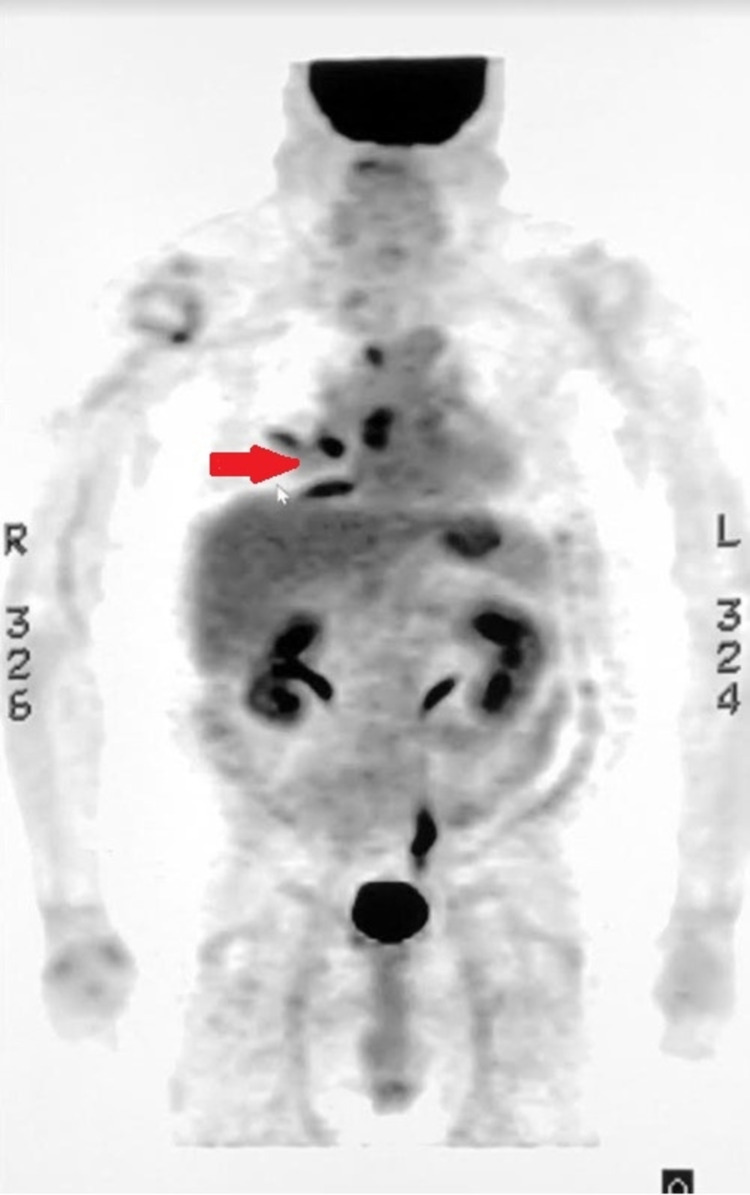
PET/CT scan which showed a large FDG avid right hilar and mediastinal lymph nodes with right paratracheal and subcarinal distribution, dense right lung middle lobe consolidation with mild medial right lung measured 1.9 X1.4 cm with SUV of 5.9
